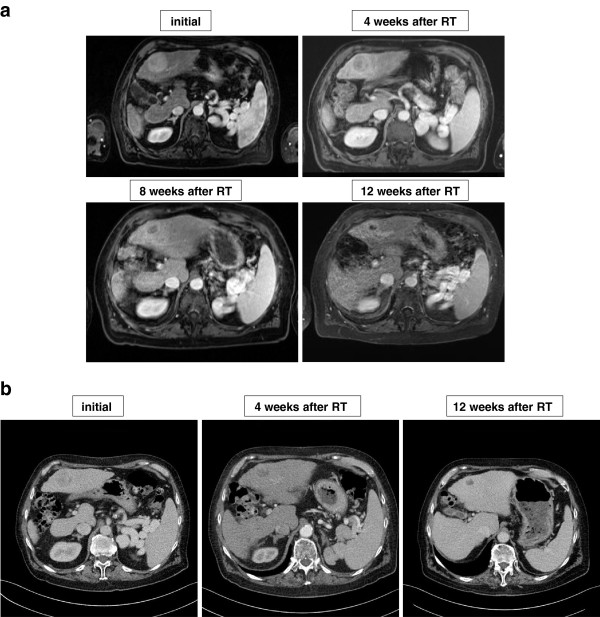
(a). Treatment response after carbon ion irradiation of a HCC lesion after 4, 8 and 12 weeks (contrast enhanced MRI). Perifocal contrast enhancement indicates radiation-induced liver reaction. (b). Treatment response of the same patient after 4 and 12 weeks (contrast enhanced CT).
